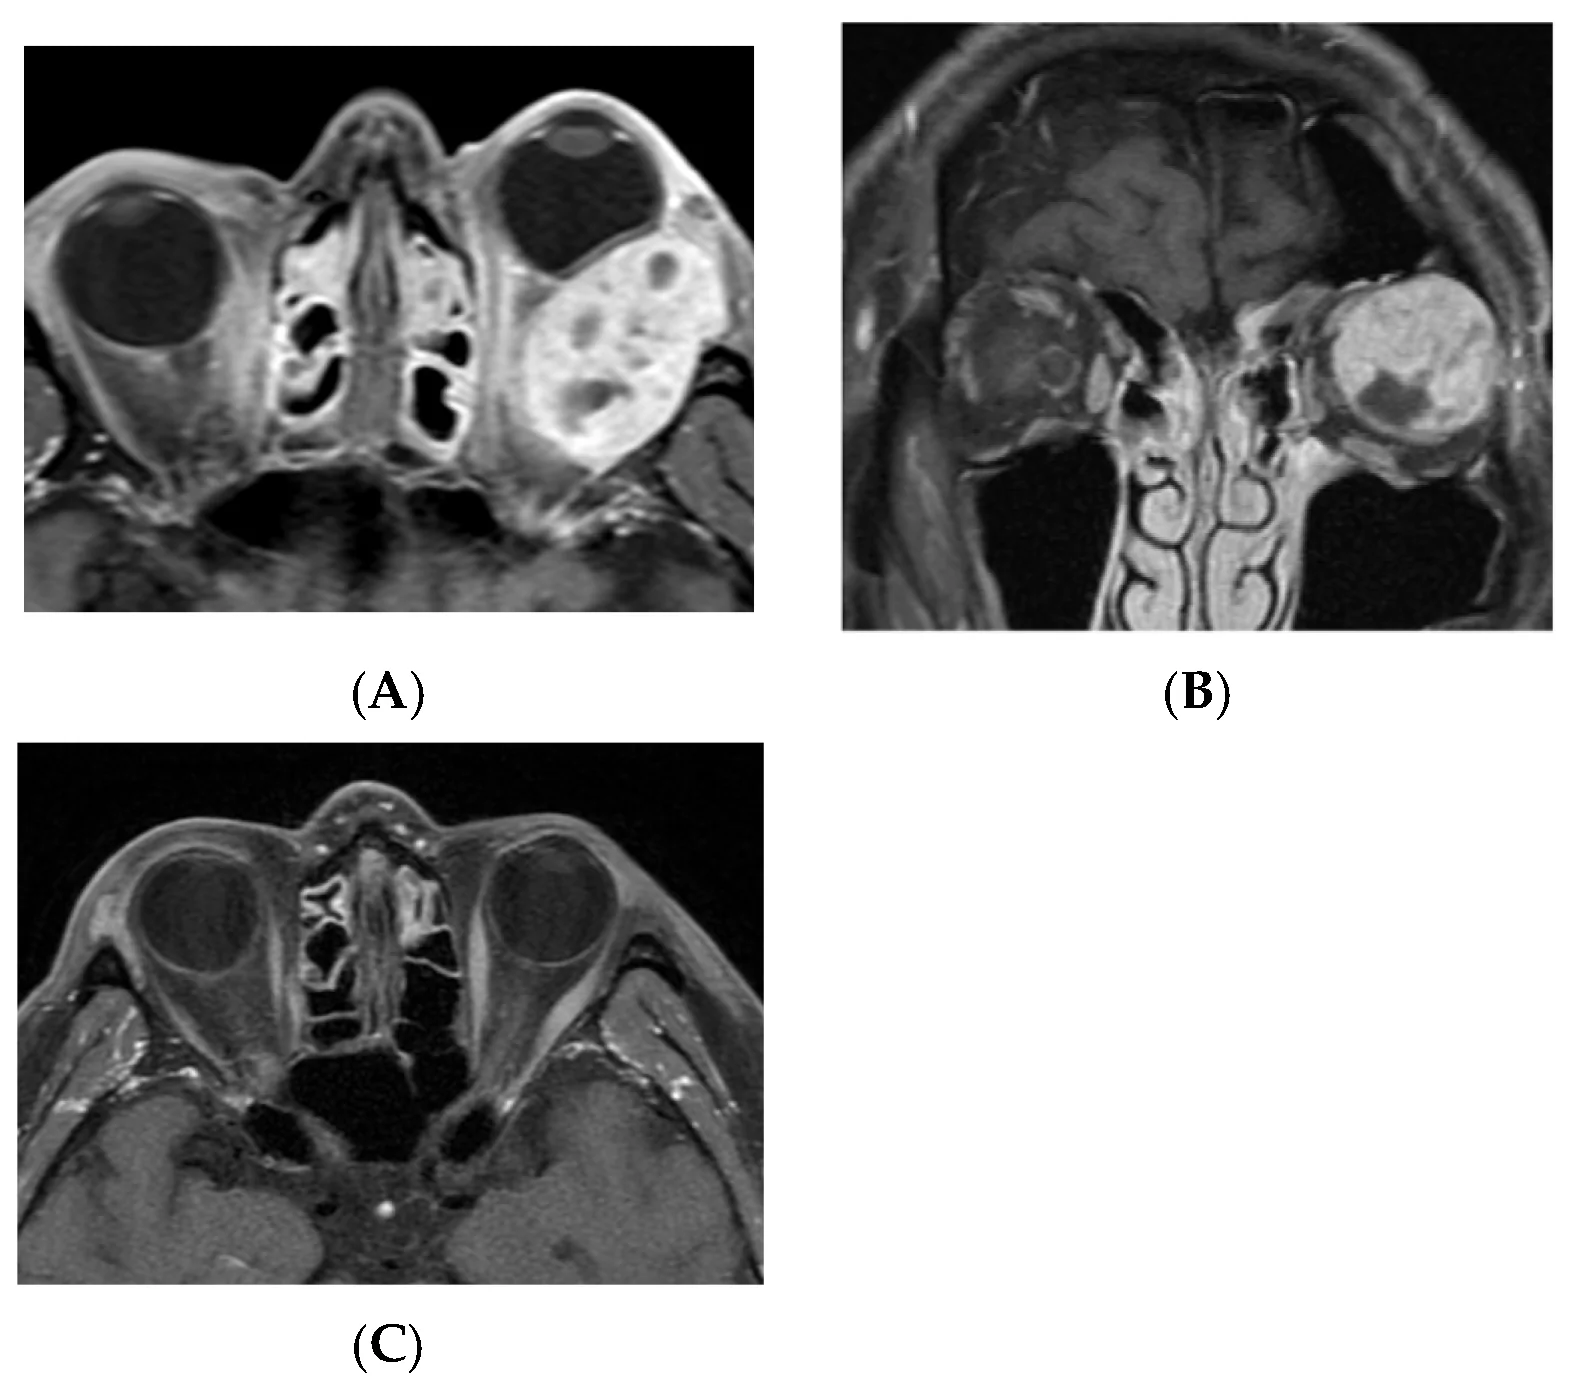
A 55-year-old man presented with a history of transient diplopia and progressive proptosis for the past 3 years with increasing “pressure sensation” in the last three months. On examination, he had 10 mm of proptosis, restriction in left gaze and some visual loss due to choroidal striae. (A,B) MRI axial (A) and coronal (B) T1 weighted post-contrast fat-suppressed images show a 3.6 × 2.9 cm mass arising from the lacrimal gland and very closely associated with the belly of the left lateral rectus muscle. There are compression and anteromedial displacement of the optic nerve and proptosis of the left eye. The patient had eye-sparing surgery: gross total resection of the lacrimal gland mass, followed by post-operative adjuvant radiation therapy. (C) MRI, 25 months after surgical resection of the lacrimal gland ACC and adjuvant radiation therapy, shows no evidence of local recurrence.
